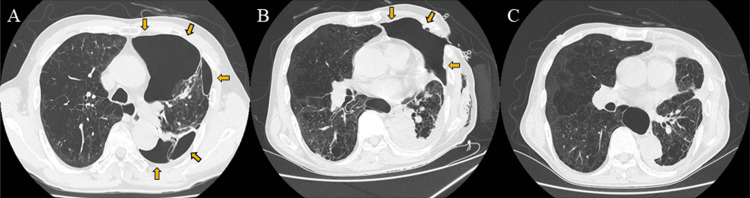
Chest computed tomography images (axial view) during hospitalization (A) Adhesion between the left upper lobe and chest wall, marked collapse of the left lower lobe, and diffuse emphysema were observed at admission. (B) Minimally invasive open-window thoracostomy using a wound protector/retractor in conjunction with three-sided taping improved the subcutaneous emphysema and resulted in left lower lung expansion. (C) Follow-up imaging showed left lung expansion and disappearance of the subcutaneous emphysema.
